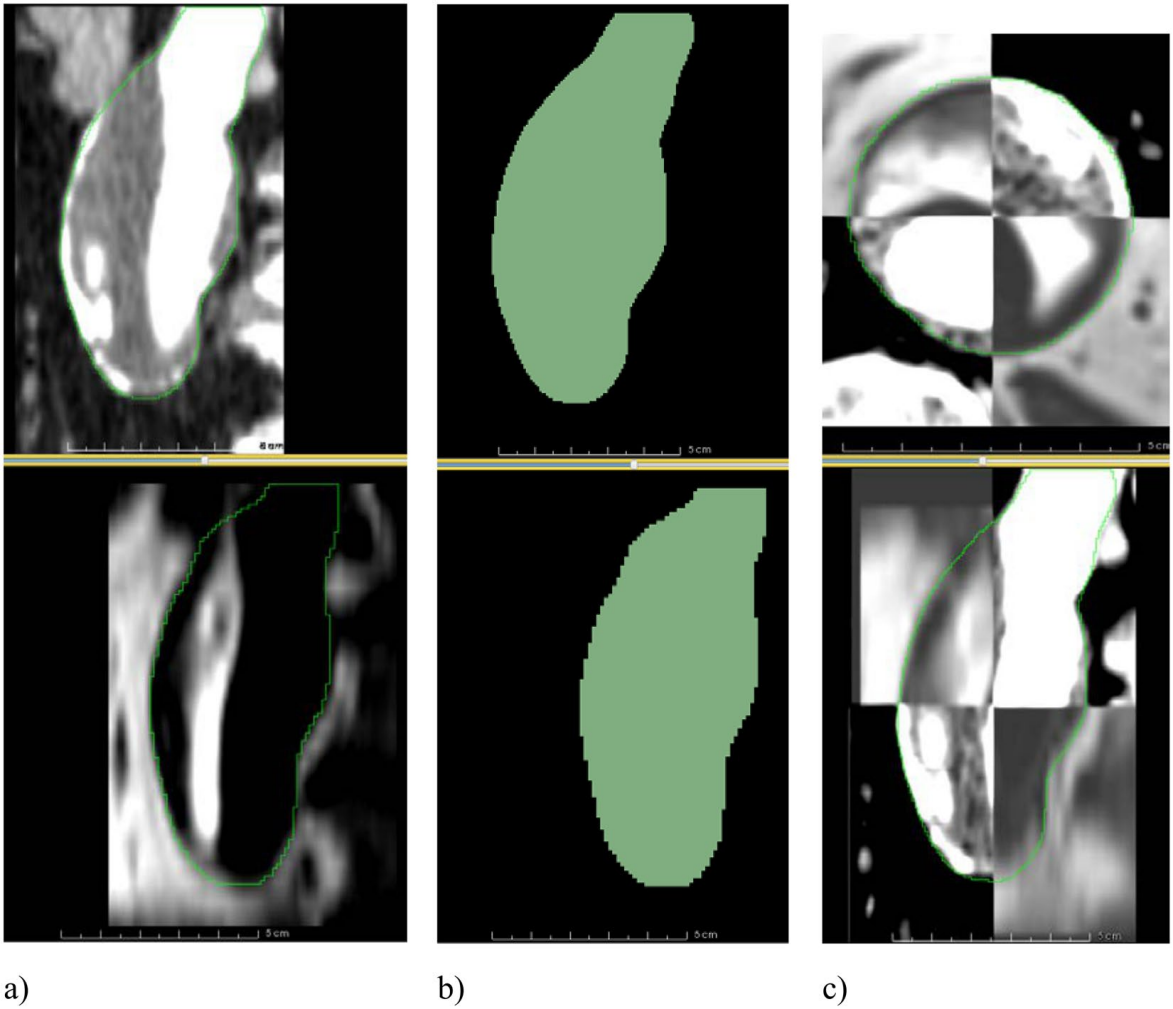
MRI to CT registration. (a) A sagittal slice of an AAA in a contrast-enhanced CT image (top) and the corresponding slice in the MR image (bottom). The contours of the AAA segmentations are shown in green. The two images occupy different positions in space and need to be registered. (b) The segmentations of the CT (top) and MR (bottom) images. The two label maps are registered and the resulting transform is saved. (c) The transform obtained from the label map registration is used to register the MRI dataset to the CT dataset. A checkerboard display of the two images is used to verify the registration result in axial (top) and sagittal (bottom) views, with the contour of the AAA segmentation shown in green.
